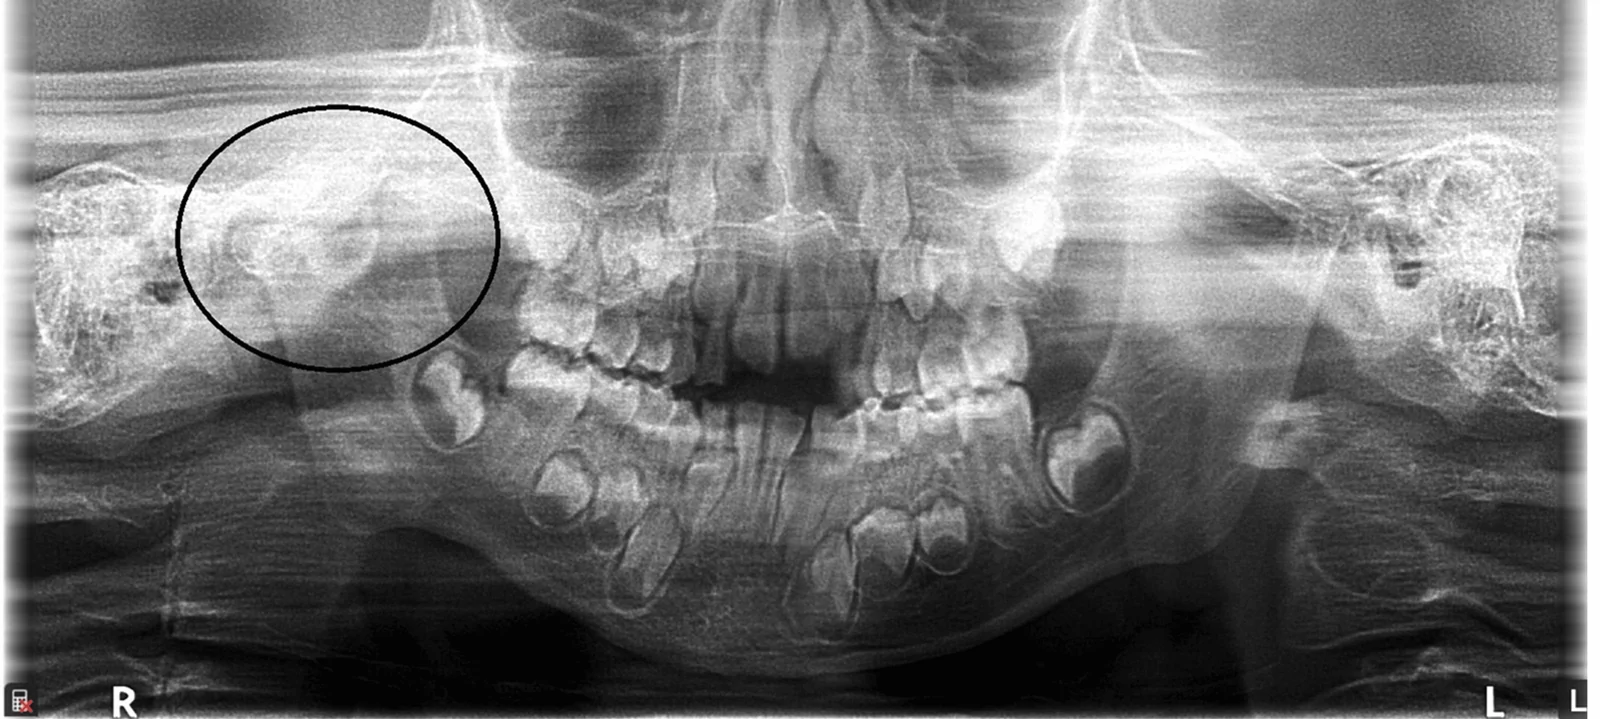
Preoperative orthopantomogram showing right temporomandibular joint bony ankylosis with obliteration of the joint space and fusion of the mandibular condyle to the temporal bone (black circle).
